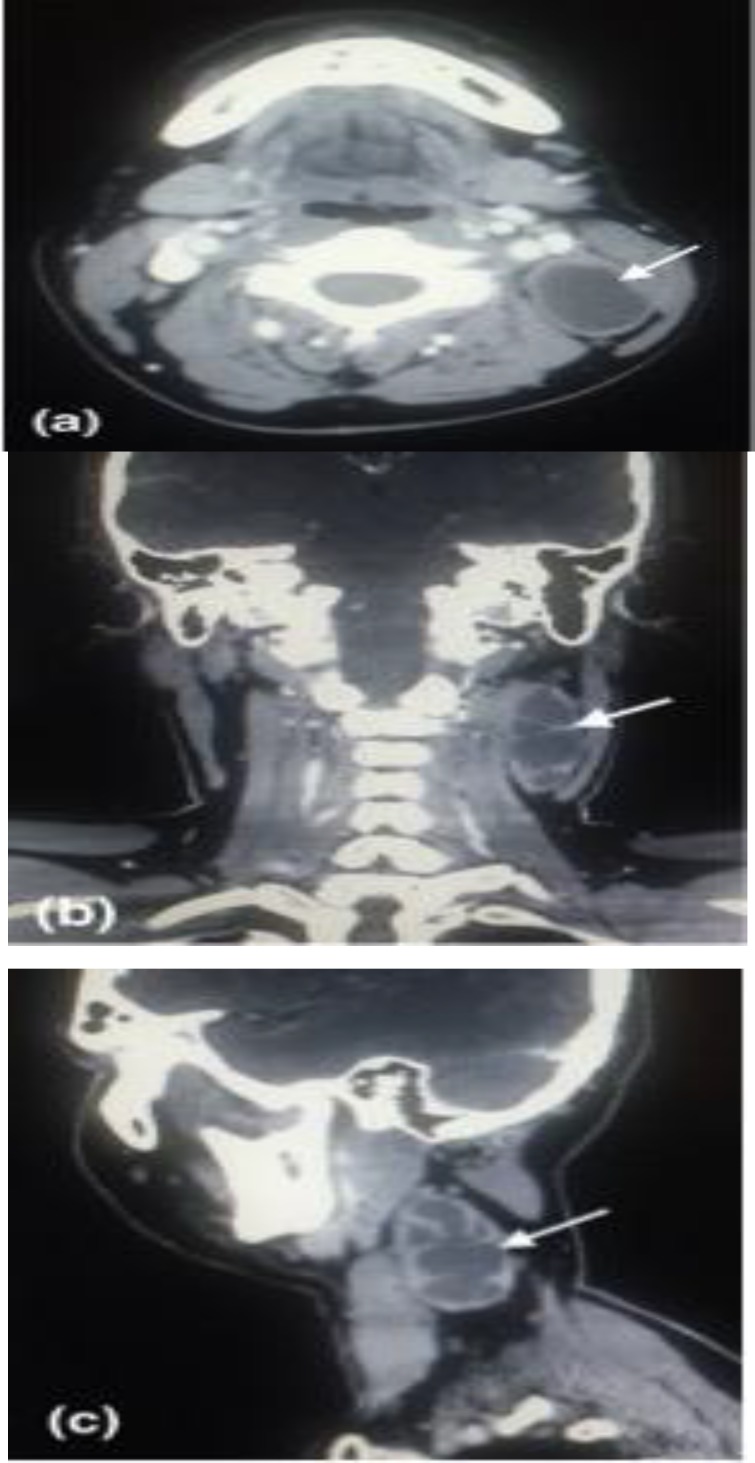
(a) Axial CT scan shows a cystic mass (white arrow) deep to left sternocleidomastoid muscle.  (b) & (c) Coronal and sagittal reconstruction CT images reveal multiple septa within the cystic mass
